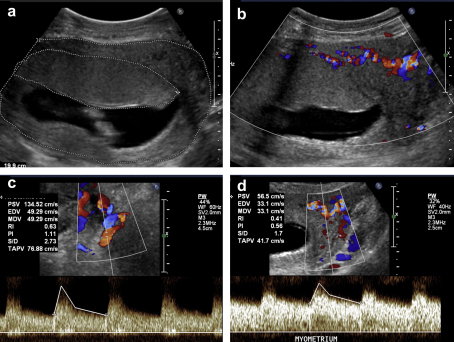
Representative real-time and colour/pulsed Doppler images of placental development at 12 weeks gestation. a) Transverse view of body of uterus outlining the uterine wall (outer line) with an anteriorly located placenta (inner line). b) The same view with colour Doppler (at conventional arterial setting of 38 cm/s). Note flow signals in this range are confined to the myometrium. c) Colour/pulsed Doppler identification of the proximal left uterine artery. Note low-impedance waveform with high (135 cm/s) peak systolic velocity. d) Colour-pulsed Doppler identification of arterial signals in the lateral myometrium, above the left proximal uterine artery. Note low-impedance waveform similar to Fig. 3c but at lower (56 cm/s) velocity. No such signals are observed entering the basal plate of the placenta. Whilst these images suggest intra-myometrial shunting at the end of the first trimester, the extent to which this phenomenon occurs in the second and third trimesters requires further research.
